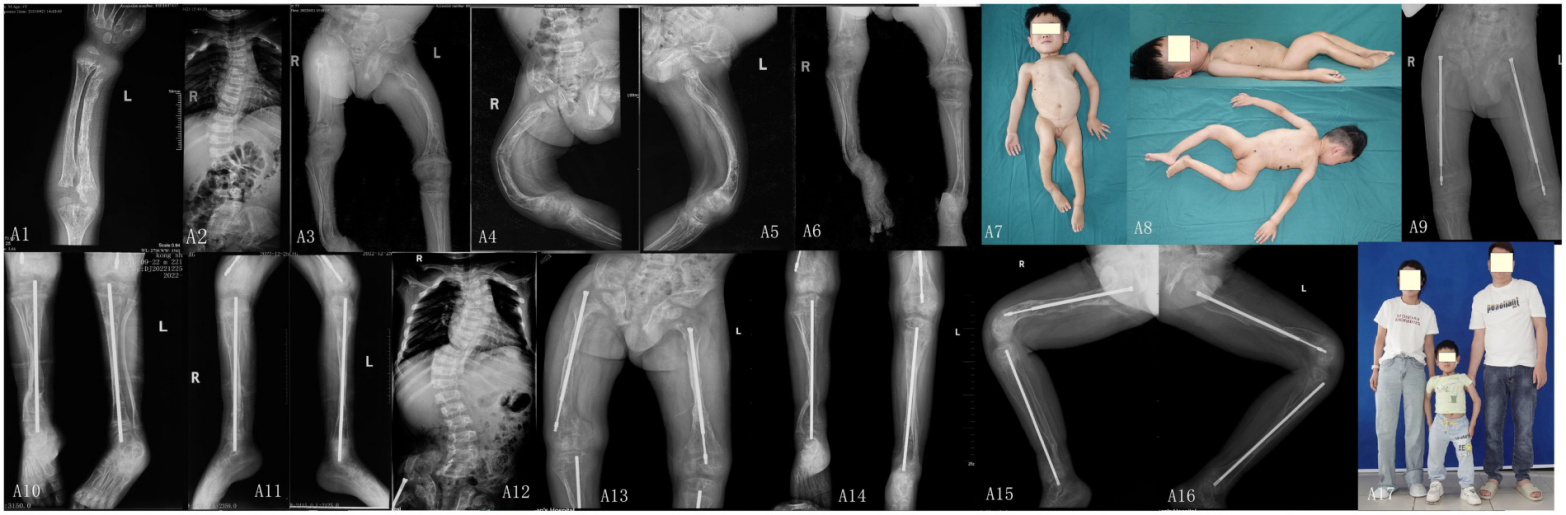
Radiographic and clinical progression of Case 1. A1. At age 5, fracture of the left radius and ulna with osteoporosis, showing a cup-shaped deformity of the radius. A2. At age 5, scoliosis with vertebral compression deformities. A3–A6. Anteroposterior and lateral views of bilateral femurs and tibiae-fibulae show multiple bone abnormalities with deformities, osteoporosis, and obvious bowing deformities of the femurs and tibia-fibula. A7, A8. Obvious deformities in the limbs, with significant bowing deformities of the bilateral femurs, tibiae-fibulae, and bilateral forearms. There are also changes in melanocytic nevi on the abdomen, back, neck, and lower jaw. A9–A11. At age 6 (1-year post-surgery), x-rays showed satisfactory correction of femoral and tibial tibia-fibula curvature with restored mechanical alignment. Fracture union at osteotomy sites and longitudinal growth of the telescopic Fassier–Duval nails are noted. A12. At age 9, progressive scoliosis with worsened vertebral compression. A13–A16. At age 9, radiographs at anteroposterior and lateral views show satisfactory fracture union with appropriate elongation of telescopic Fassier–Duval nails and improved bone density. A17. Assisted standing with persistent right limb shortening and residual forearm deformities.
